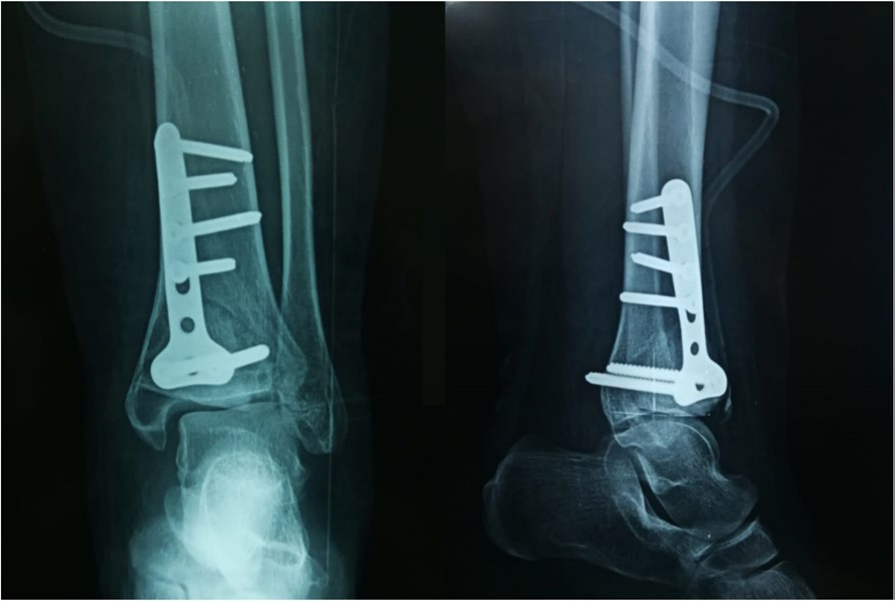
Immediate postoperative anteroposterior and lateral X-rays
